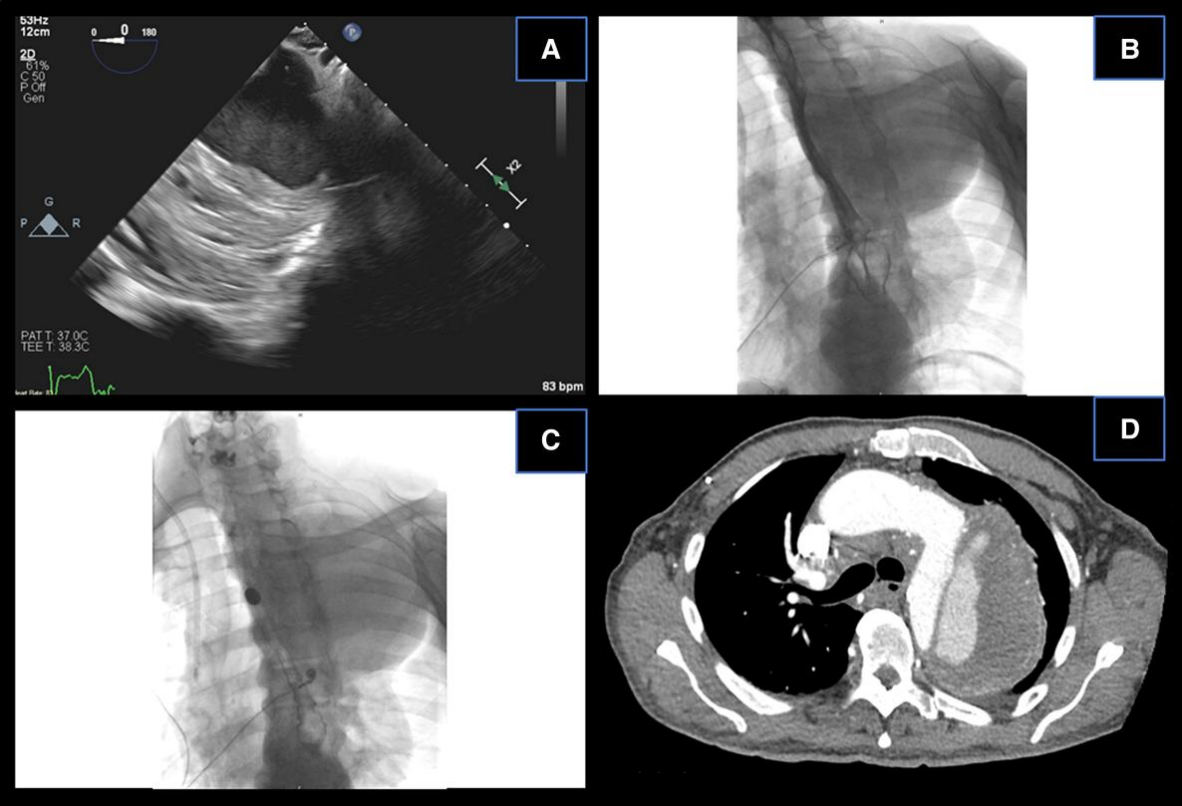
(*A*) A large 9.6 cm dissection of the aortic arch and proximal descending aorta with thrombosis of the false lumen as visualized on transoesophageal echocardiography. (*B*) Barium oesophagram demonstrating a large aortic aneurysm (dark silhouette in the upper left hemithorax) compressing the oesophagus at the T5 level. (*C*) Administration of a 13 mm barium tablet, trapped at the level T5 (level of oesophageal compression by the aortic aneurysm). (*D*) Computed tomography and angiography with a large DeBakey type III aortic dissection and partial thrombosis of the false lumen in close proximity to the oesophagus.
